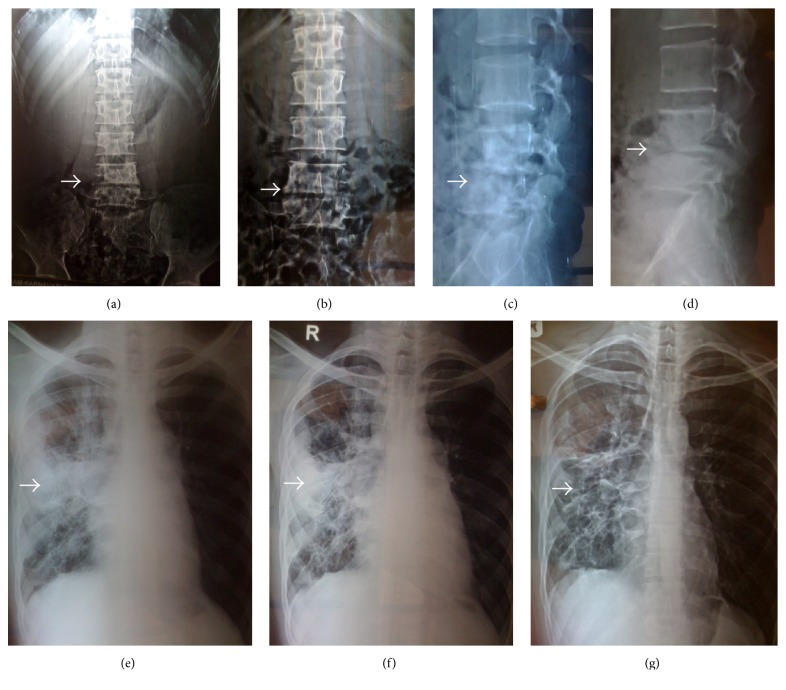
Radiographic evidence of improvement in spine and chest after antifungal treatment. (a) and (b) represent anteroposterior X-rays of lumbar spine, before and after treatment, respectively. (c) and (d) represent lateral X-rays of lumbar spine, before and after treatment, respectively. (e), (f), and (g) represent the sequential improvement in chest radiographs after the initiation of antifungal treatment.
